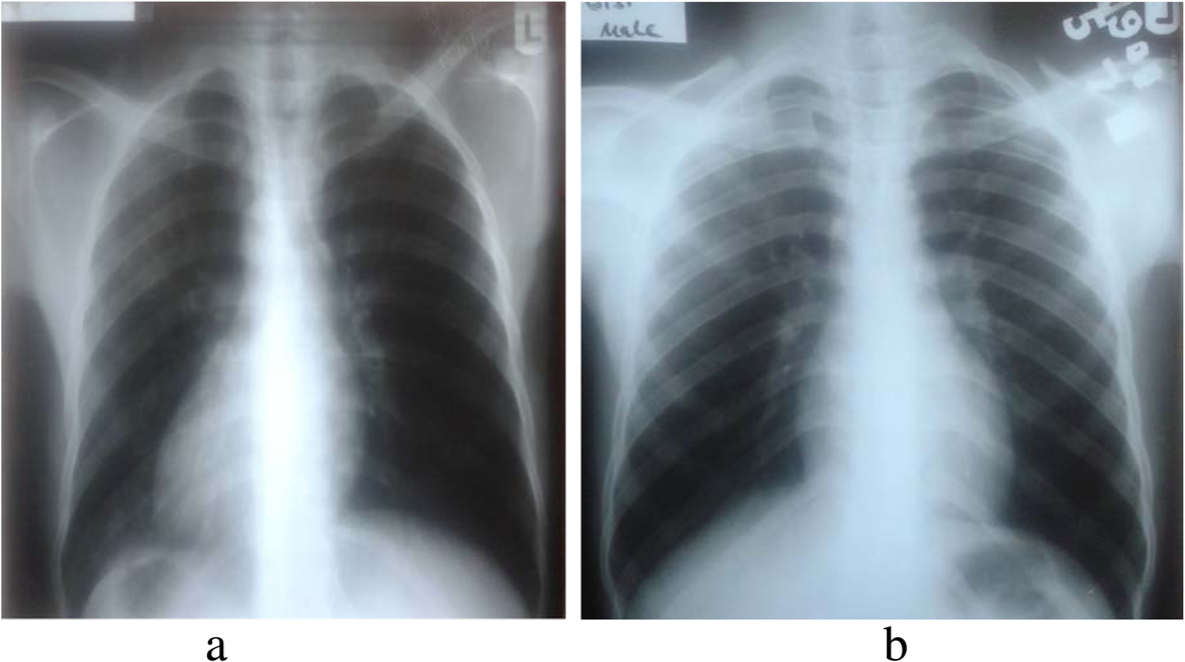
Chest X-ray of a 22-year-old Nigerian man with dextrocardia and situs inversus (a) compared with a chest X-ray of a 20-year-old Nigerian man (b) with the heart and abdominal organs normally positioned
